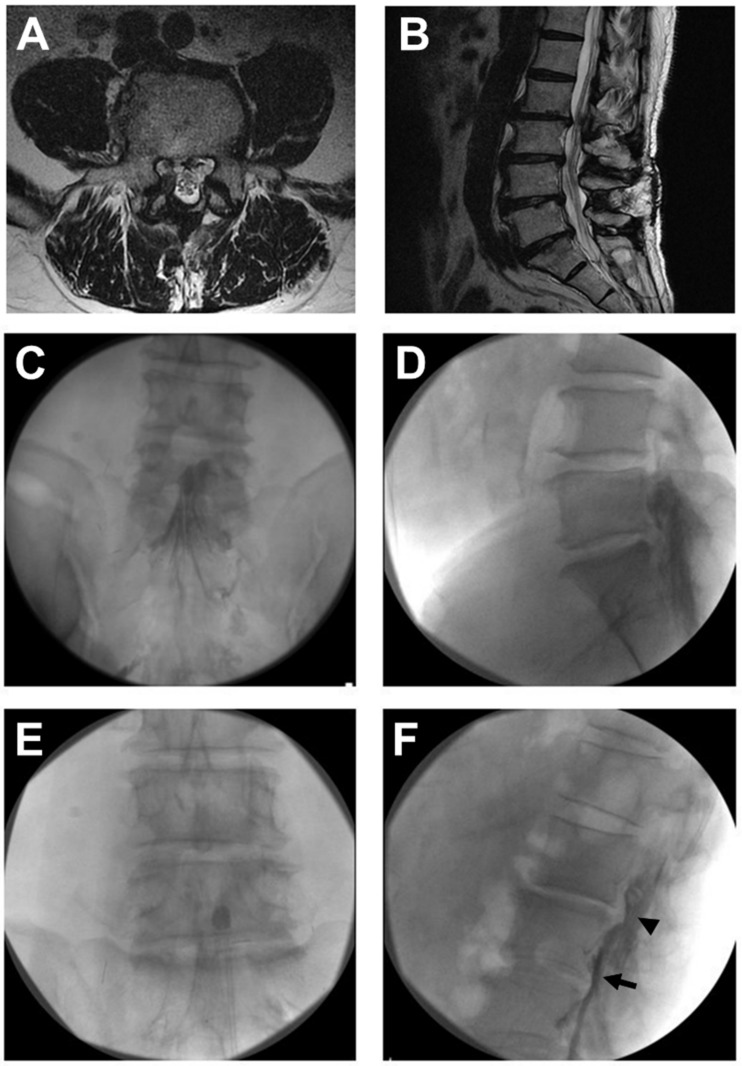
Percutaneous epidural adhesiolysis combined with balloon decompression in a patient with lumbar post-laminectomy syndrome. A 61-year-old male with a history of L4-5 partial laminectomy and flavectomy 1 year prior underwent combined epidural adhesiolysis and balloon decompression. (A) Adhesive cauda equina is shown in a cross-sectional magnetic resonance imaging (MRI) image at L4-5 level. (B) A sagittal MRI image of identical patient shows well-decompressed state in L4-5 level. (C) Anteroposterior fluoroscopic view showing a definite contrast filling defect in the previously operated L4-5 level. (D) A lateral fluoroscopic image showing a filling defect above the L4-5 level. (E) The inflatable balloon catheter placed in the suspected adhesion site and balloon filled with the contrast medium. (F) Contrast flow detected above the L4-5 level after the balloon procedure (arrowhead). The catheter is noted (arrow) in the anterior epidural space.
